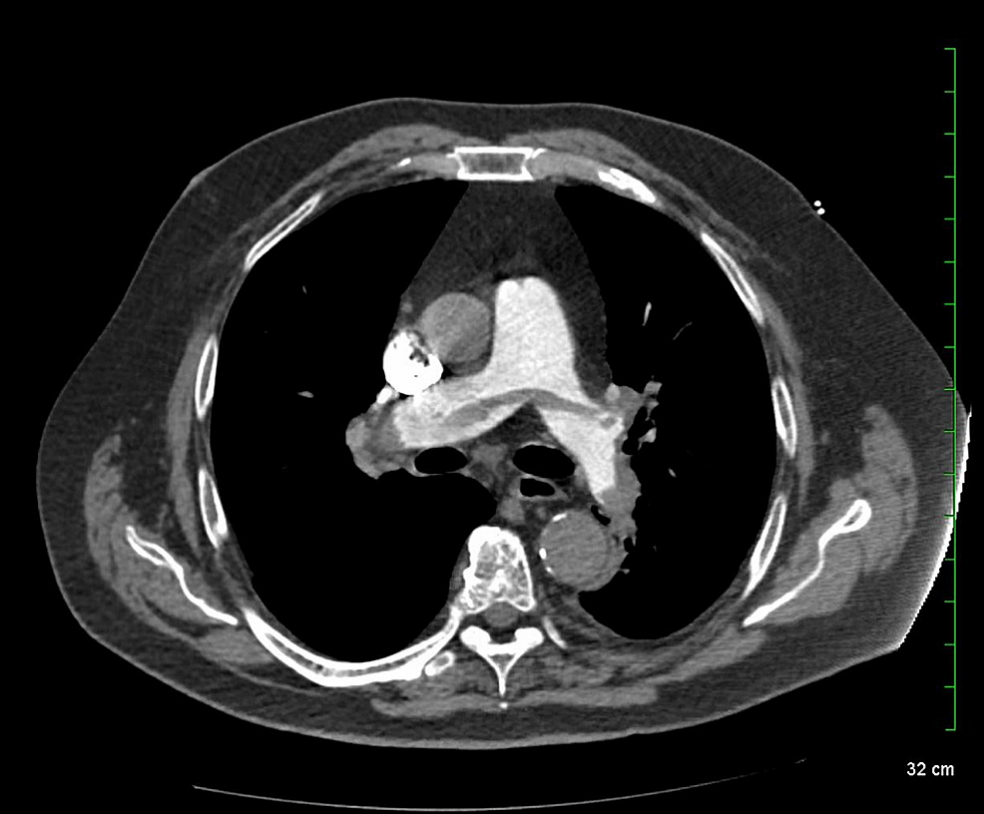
CT-pulmonary embolus protocol: saddle embolus with bilateral occlusive and non-occlusive emboli in both the right and left branches of the pulmonary artery
